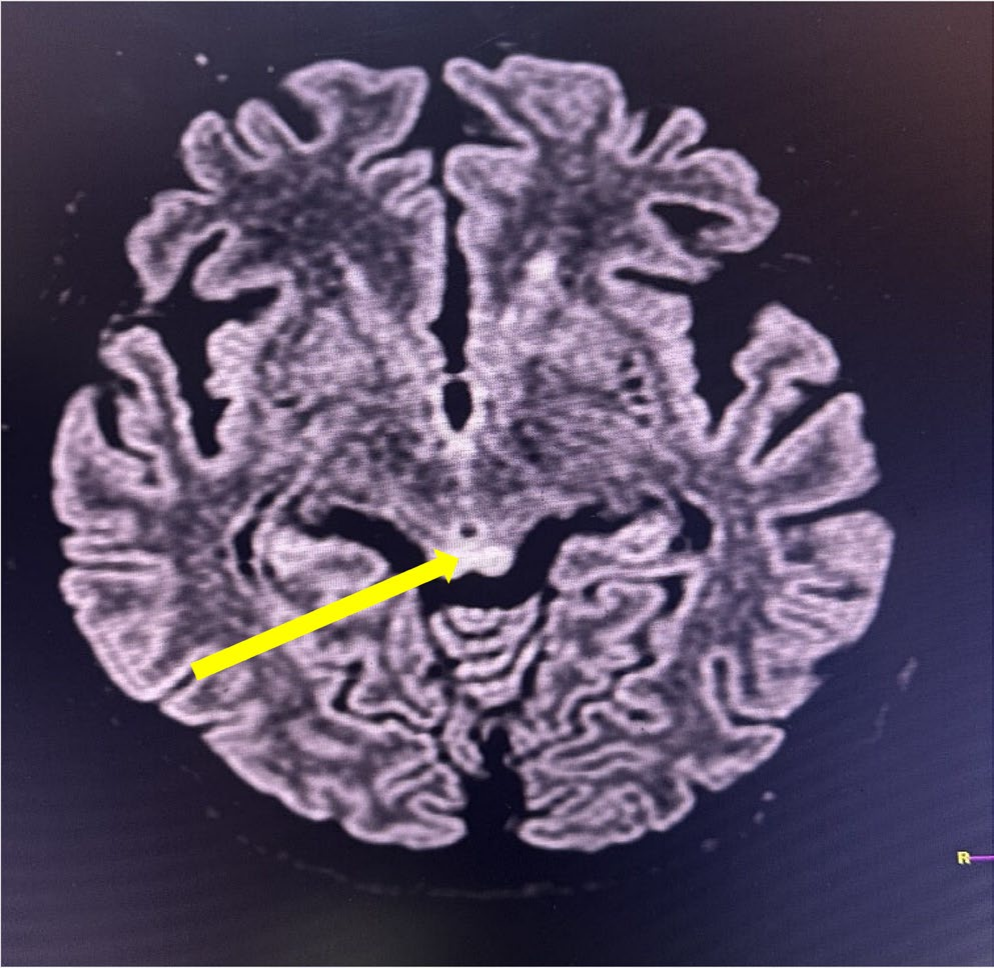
Cerebral MRI T2/FLAIR hyperintensity involving bilateral periaqueductal gray matter (yellow arrow), suggestive of WE.
